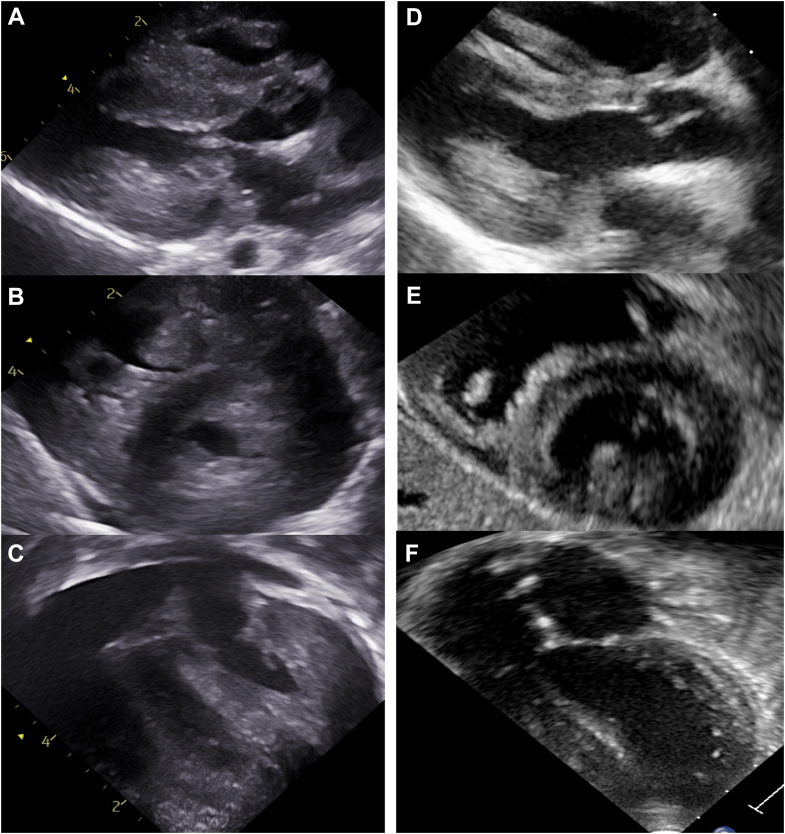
Echocardiographic Imaging at Admission and Discharge (A-C) Admission anatomy from parasternal long (A) and short (B) axis, and apical 4-chamber view (C). (D-F) Improvement in ventricular hypertrophy from corresponding views before discharge (after 9 months of MEK inhibition).
